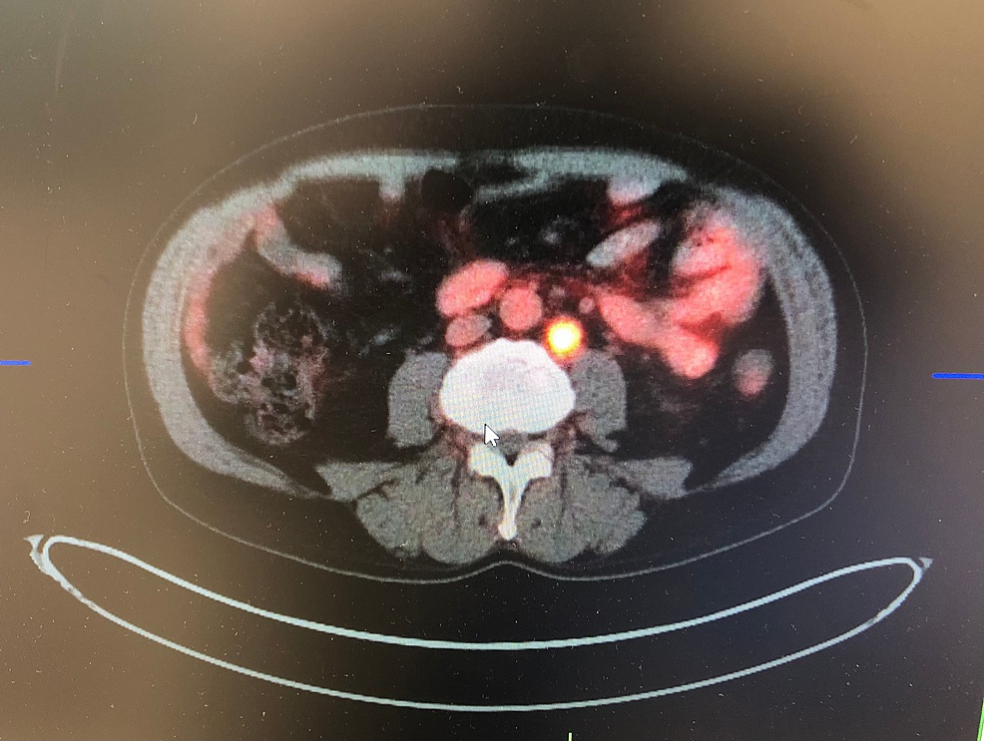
Follow-up PET CT scan 20 weeks after complete remission of the lesions showed hypermetabolic right para-aortic, left retroperitoneal, and right retrocrural lymph nodes.
